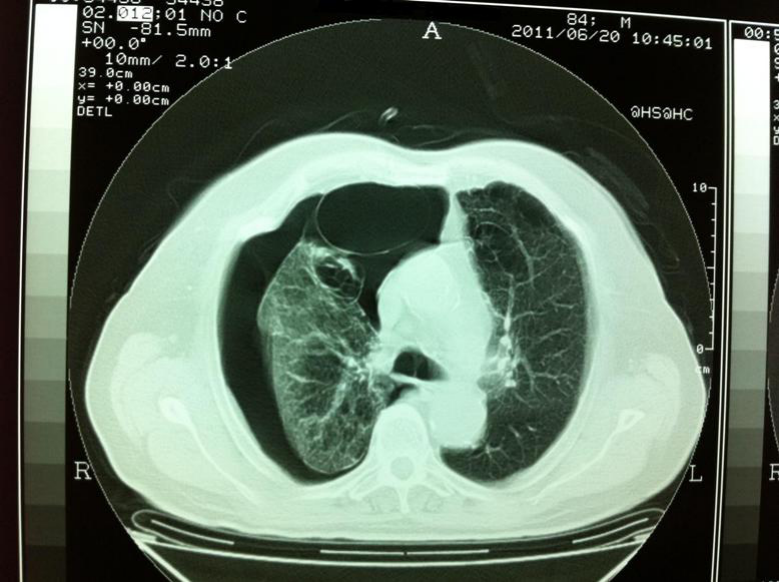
Preoperative CT scan image of the patient (lung window) which shows the extend of bullous disease.
